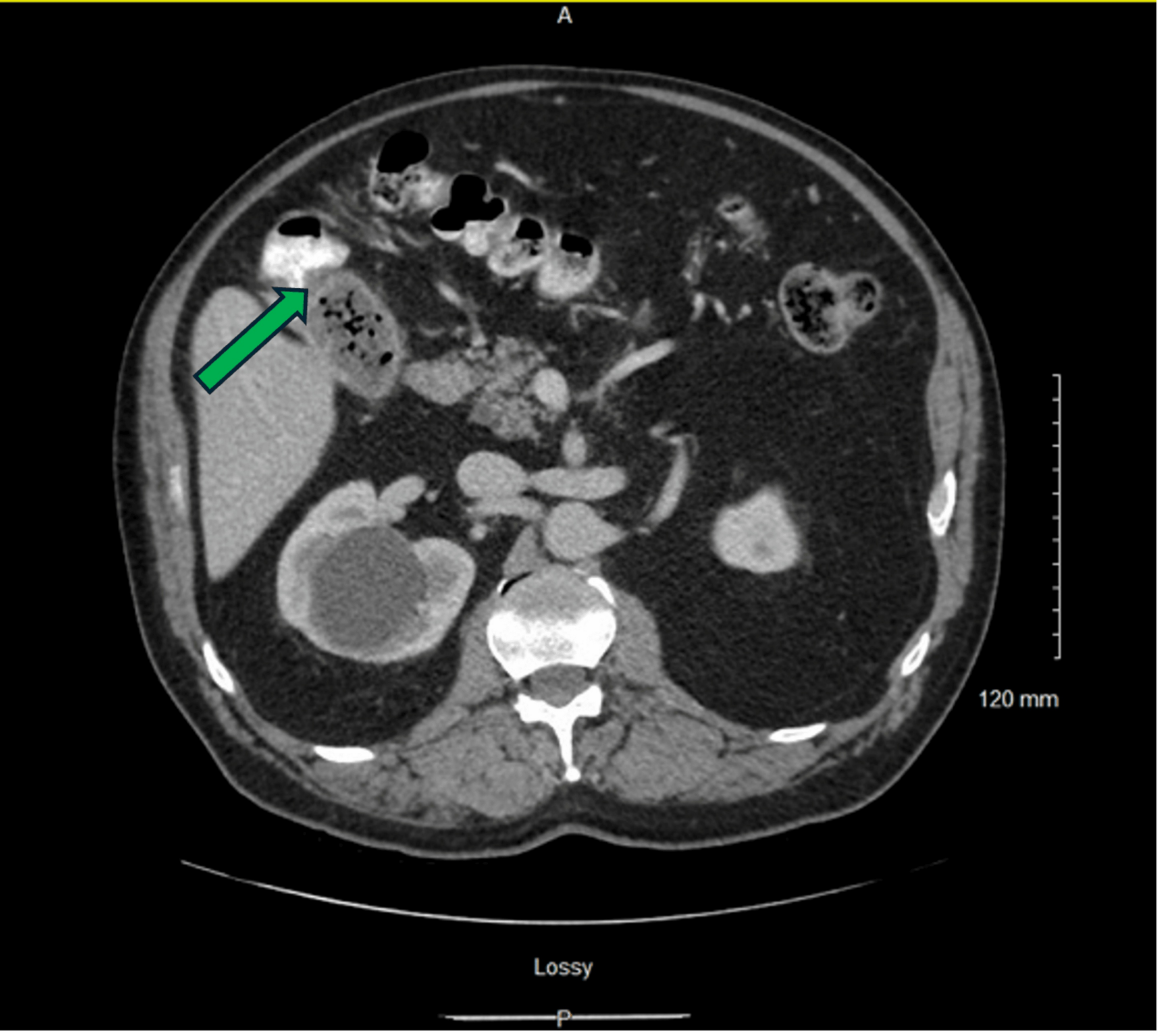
Axial computed tomography image with intravenous contrast demonstrating the fistula with associated pneumobilia.
